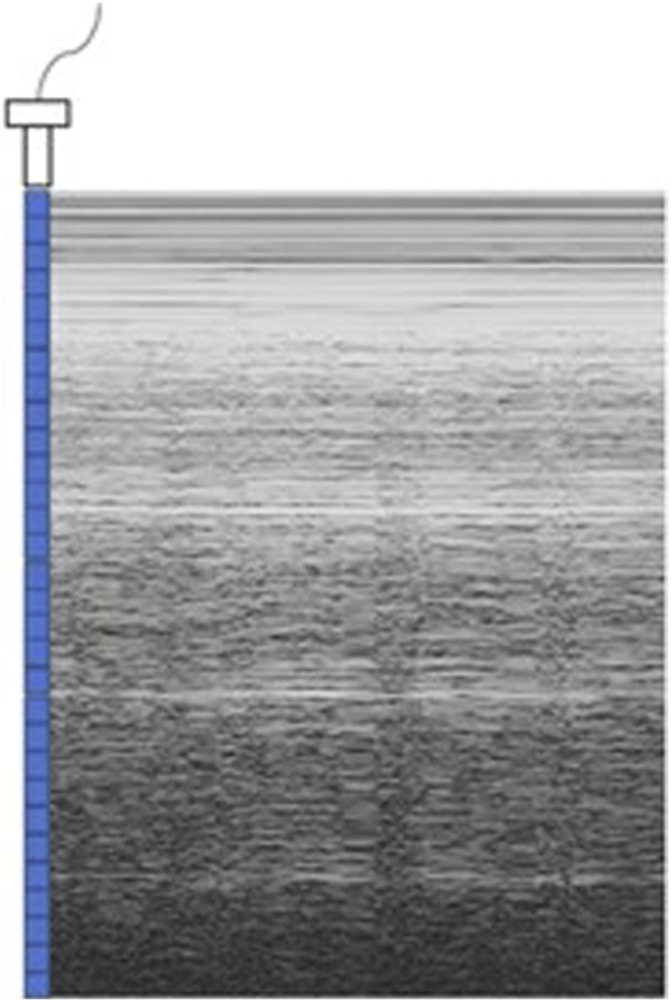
M-type ultrasound image.
